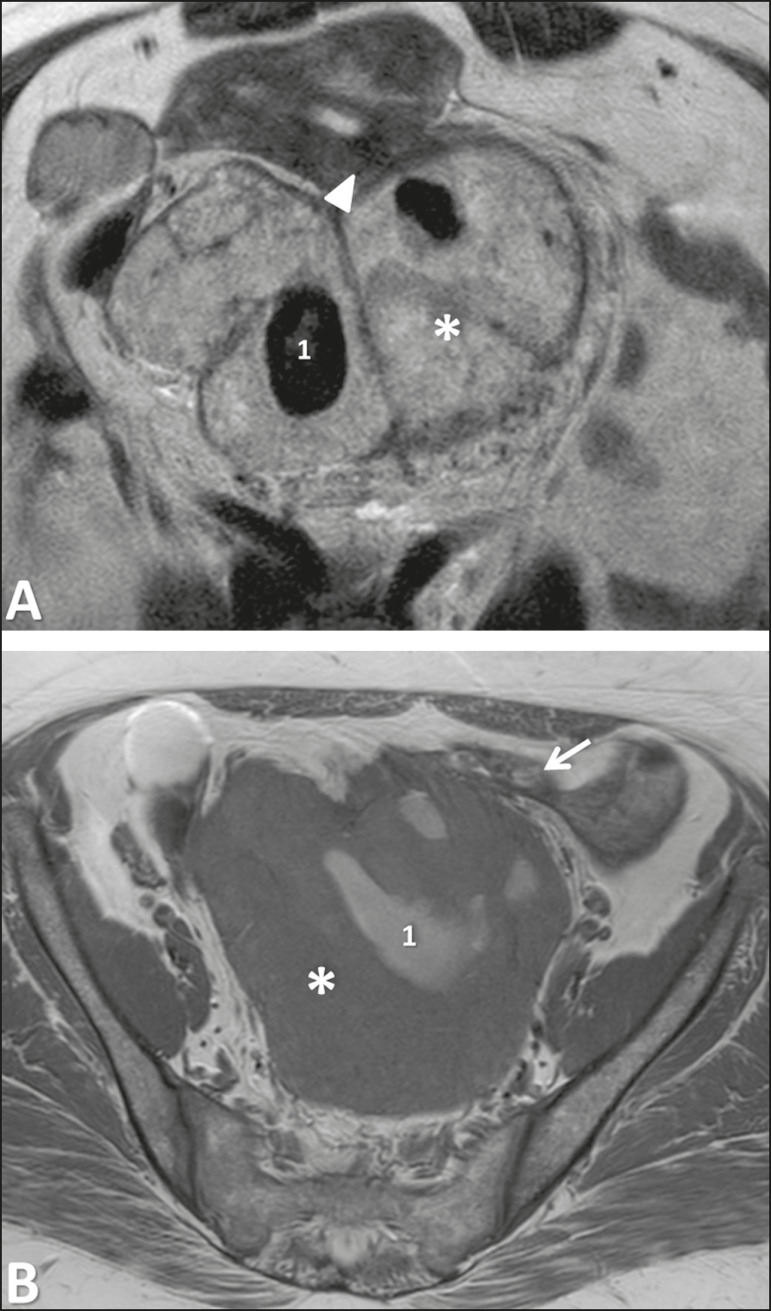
Endometriosis of the broad ligament in a 49-year-old woman. Axial T2-weighted imaging (A) and axial T1-weighted imaging (B) showing a heterogeneous tumor (asterisk), with internal areas with high T1 signal compatible with hemorrhagic areas (1), demarked from the left ovary (arrow) and the uterus (triangle).
